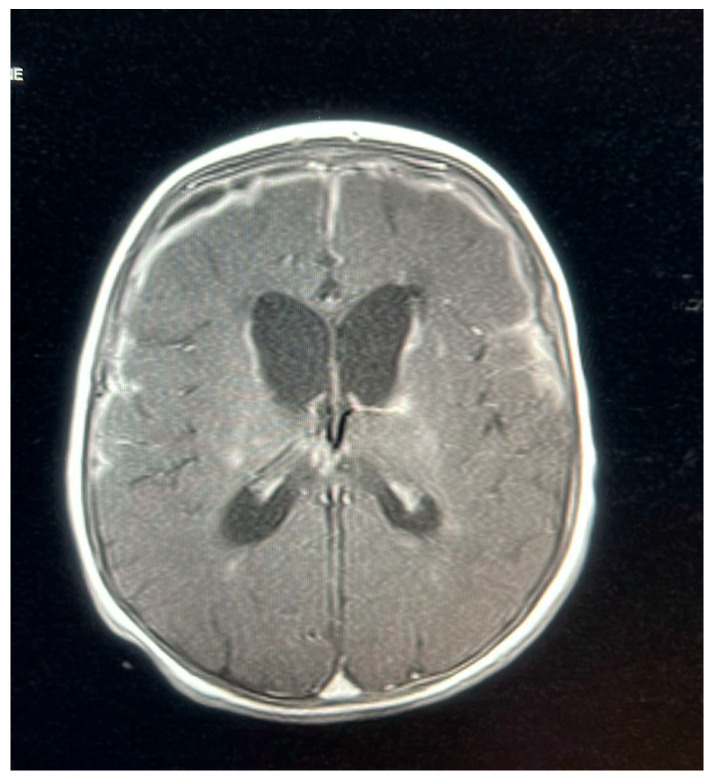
MRI brain scan showing worsening of leptomeningeal enhancement.
